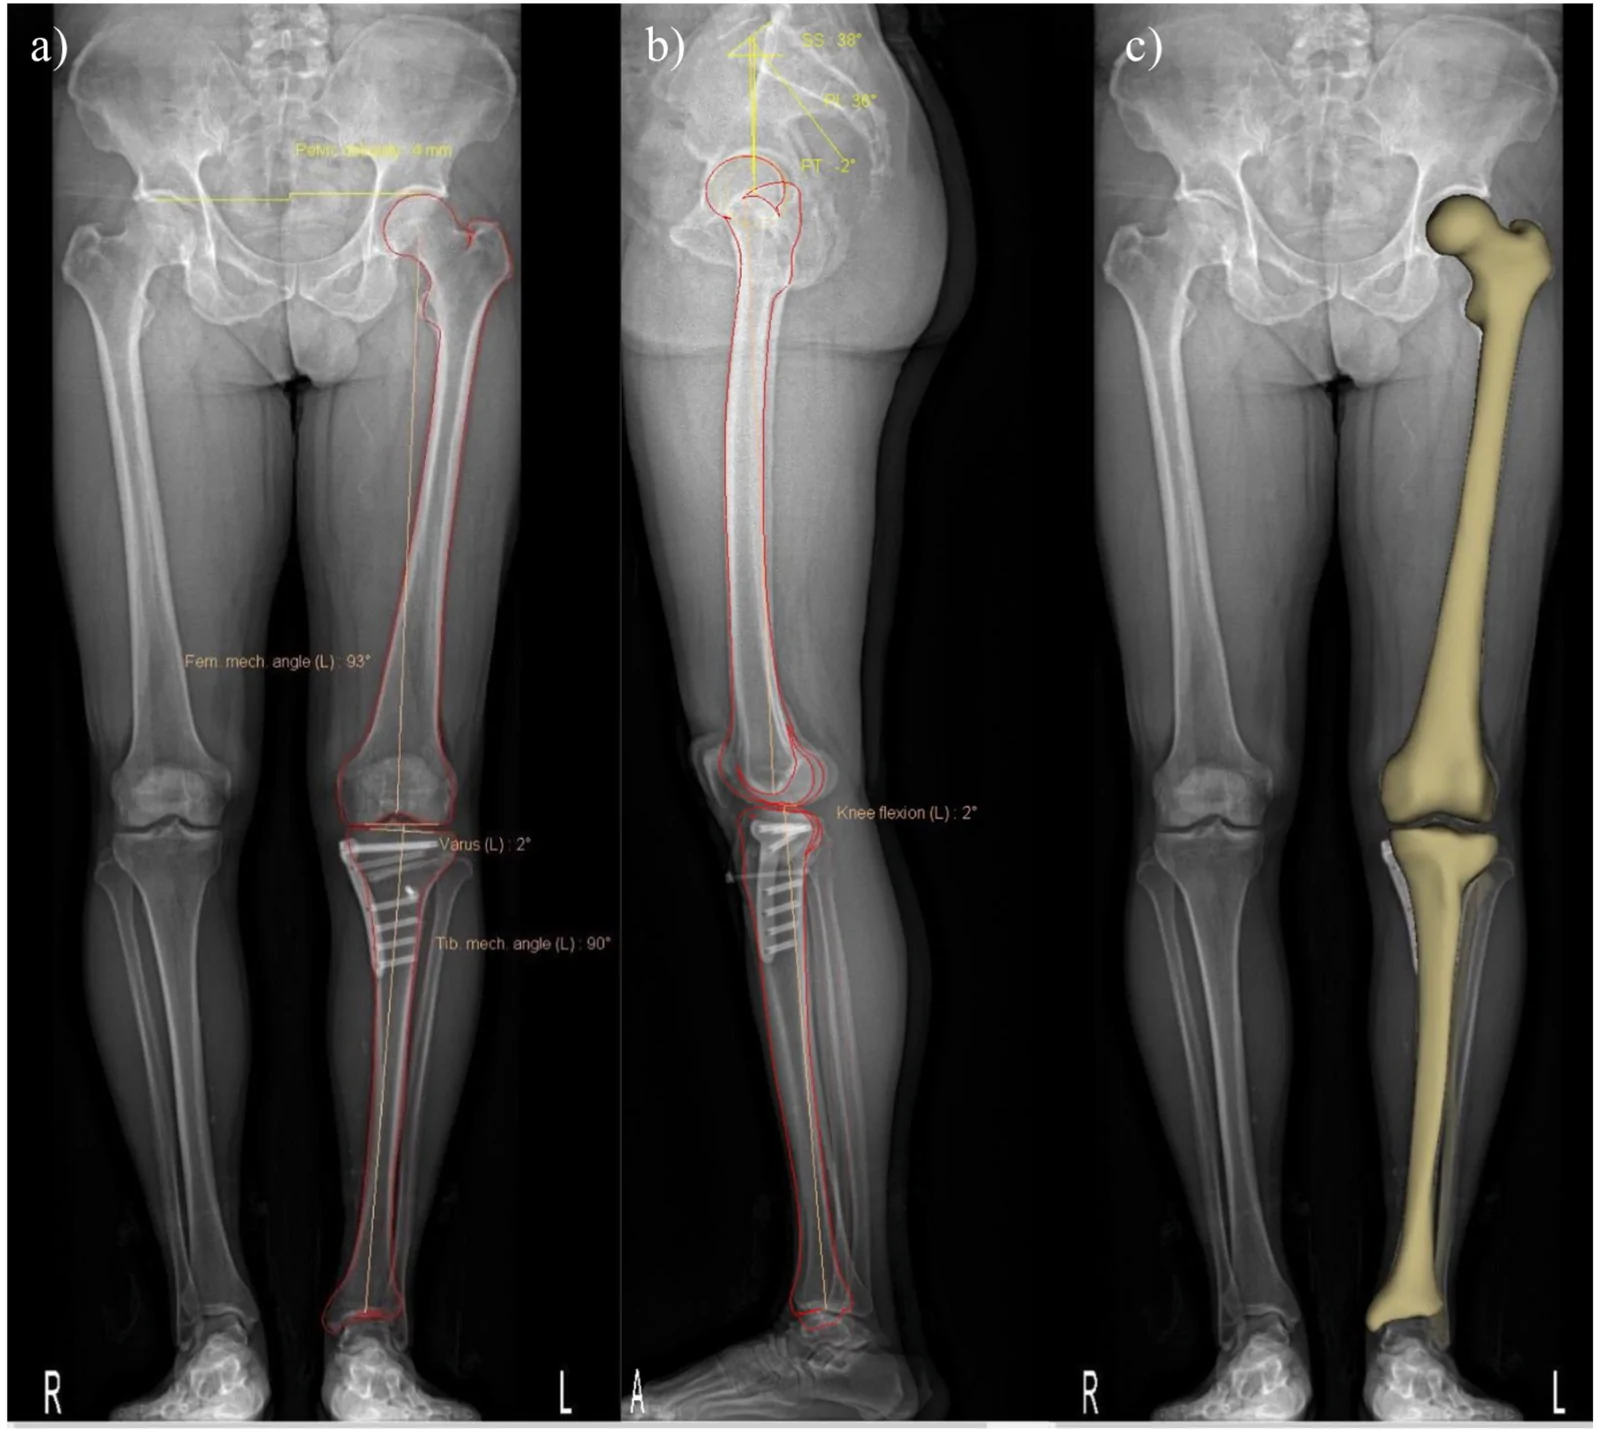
Radiographic measurements of lower limb alignment and spinopelvic parameters after open wedge distal tibial osteotomy using the EOS system (a) Coronal alignment was assessed based on spinopelvic parameters, including pelvic obliquity, and lower limb alignment. Knee varus and valgus were defined using the coronal hip–knee–ankle angle (cHKA), while additional alignment measures included the mechanical lateral distal femoral angle (mLDFA) and the medial proximal tibial angle (MPTA). (b) Sagittal alignment was assessed based on spinopelvic parameters– pelvic incidence (PI), sacral slope (SS), pelvic tilt (PT)-as well as lower limb alignment including the sagittal hip-knee-angle (s-HKA). (c) 3D reconstructed models using the sterEOS workstation.
